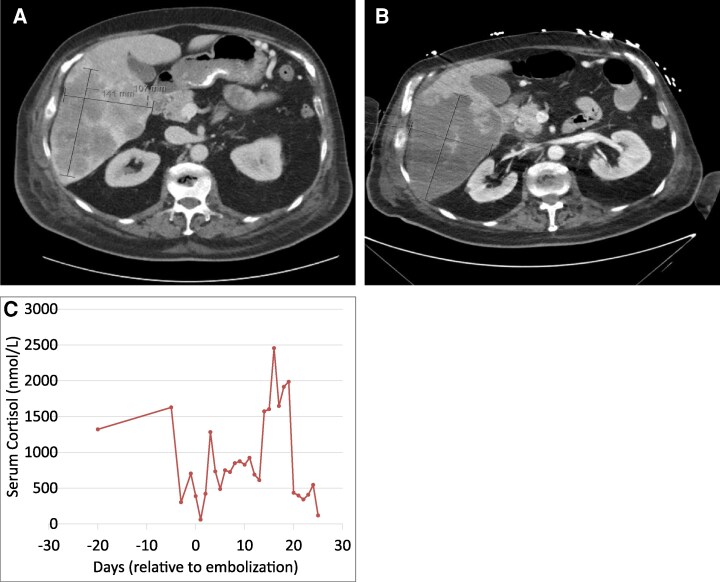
Short-term imaging follow-up after bland embolization in case 3. Axial contrast-enhanced computed tomography images demonstrating a dominant heterogeneous hepatic mass in the right hepatic lobe prior to (A) and 2 weeks after (B) bland embolization. The lesion measured 14.1 × 10.7 cm pretreatment (A) and 14.9 × 10.0 cm on early follow-up imaging (B), without significant size reduction. Minimal biochemical response observed following bland hepatic embolization in Case 3 (C).
